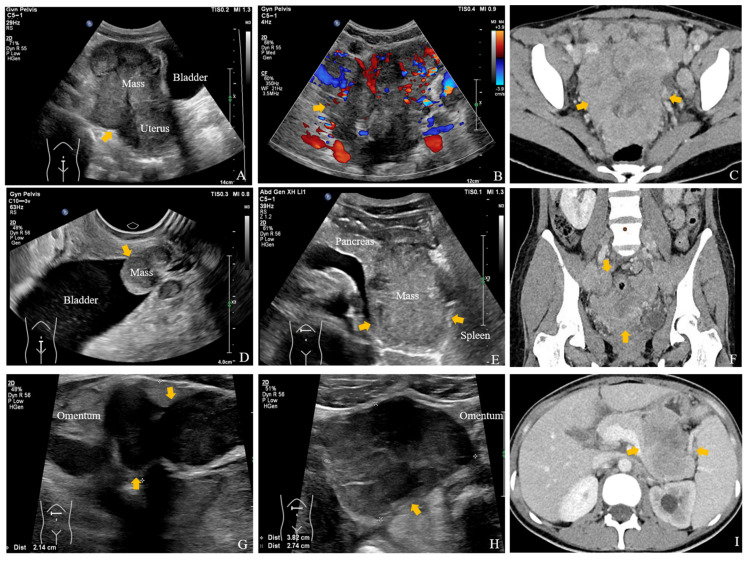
US and Contrast-enhanced CT images show metastatic lesions involving the pelvic cavity, pancreatic-splenic hilum region, peritoneum and omentum. Pelvic ultrasonography disclosed an 11.0 × 7.6 × 9.5 cm infiltrative mass involving uterus and bladder (arrow), with prominent vascular signals (arrow) (A,B). Contrast-enhanced CT showed an ill-defined heterogeneous mass in the bilateral adnexal areas (arrow) (C,F). Additional findings included a 1.9 × 1.6 cm vascularized nodule on the bladder wall (D), arrow), an 8.7 × 6.2 × 6.5 cm poorly demarcated mass in the pancreatic-splenic hilum region on ultrasonography (E), arrow) and contrast-enhanced CT (I), arrow), and diffuse peritoneal and omental thickening with multiple nodules (arrow), the largest measuring 3.8 × 2.7 cm (arrow) (G,H). The pelvic mass was consistent with a Krukenberg tumor, typically appearing as a heterogeneous solid lesion with potential cystic areas [1]. The irregular peritoneal thickening and omental cake appearance indicated metastatic spread [2]. The patient received seven cycles of a modified BEMA regimen combined with nivolumab. Initial treatment response was stable disease; however, follow-up showed progression at these sites, and the patient ultimately succumbed to acute obstructive renal failure due to tumor progression, which aligns with the aggressive nature and poor prognosis of advanced SRCC [3,4]. Systemic ultrasonography, combining high-frequency imaging and microvascular techniques, plays a crucial role in detecting rare metastatic sites and monitoring therapeutic response in real-time, offering an early, sensitive, radiation-free, and repeatable assessment for patients with poor general condition [5,6].
